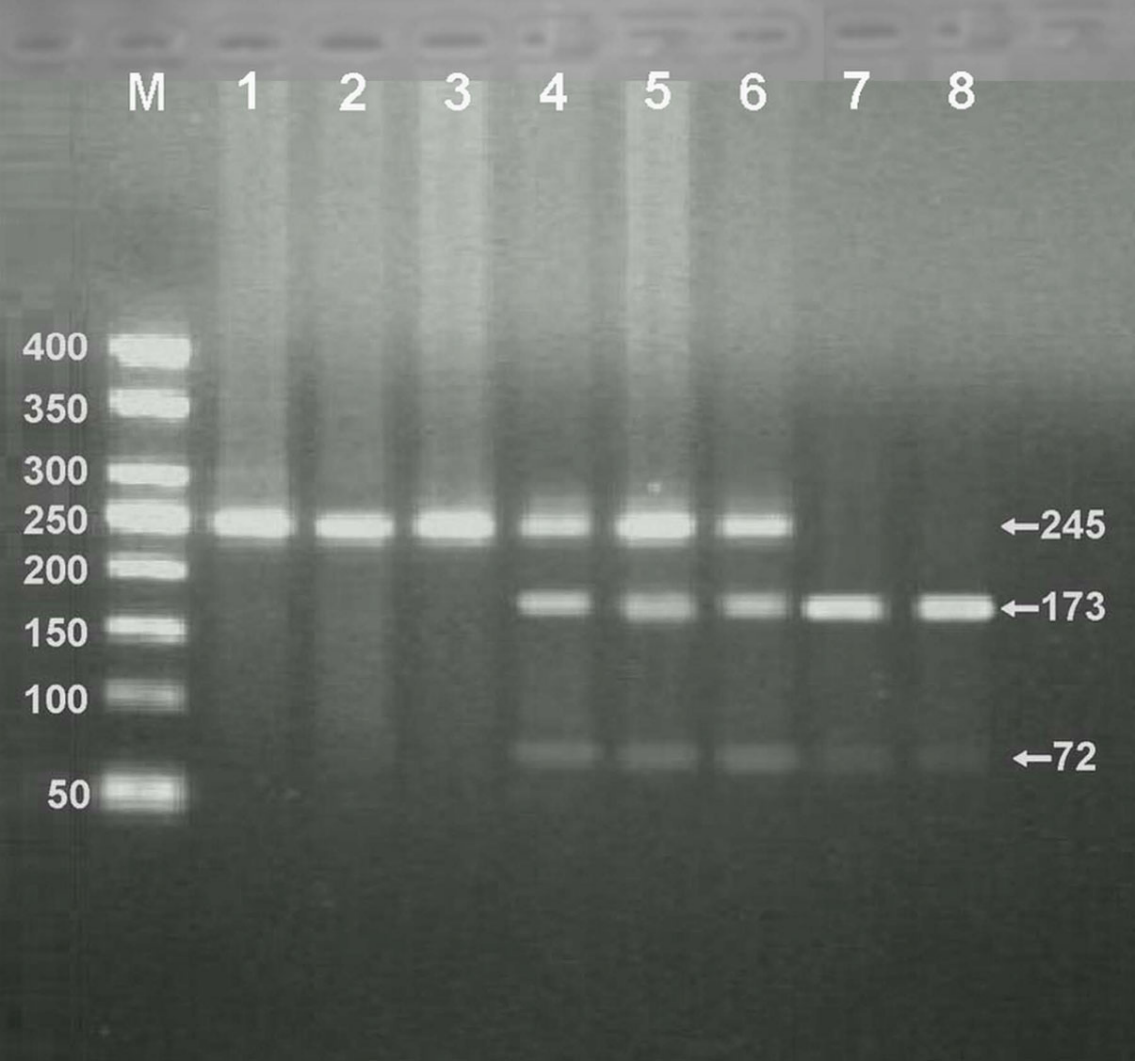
Genotyping of the MTHFR C677T polymorphism. Lane M, 50 bp marker ladder; lanes 1 and 2, CC genotype (245 bp); lane 3 and 4, CT genotype (245-, 173- and 72-bp); and lanes 5 and 6, TT genotype (173- and 72-bp).
